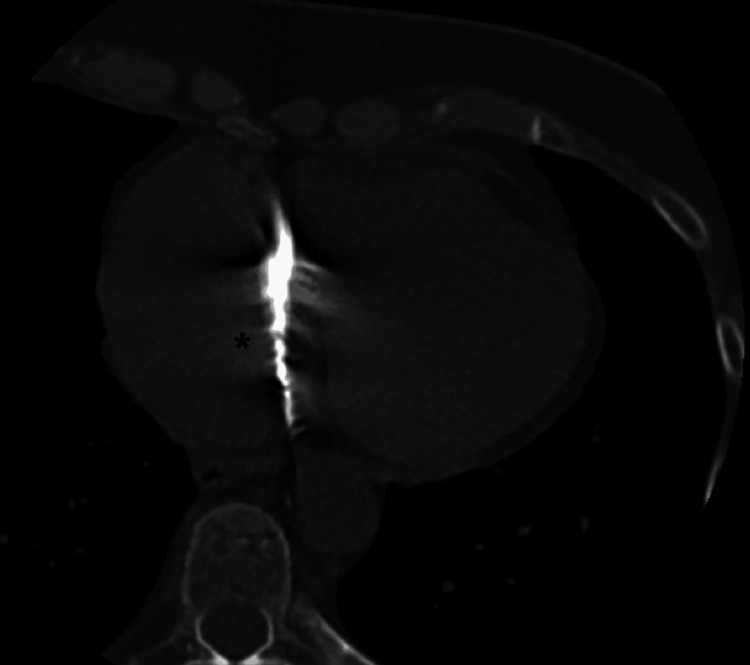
CT scan of the heart (coronal cut) showing the cardiac structure (asterisk) with the same density as the injected and infiltrated material CT: computed tomography
